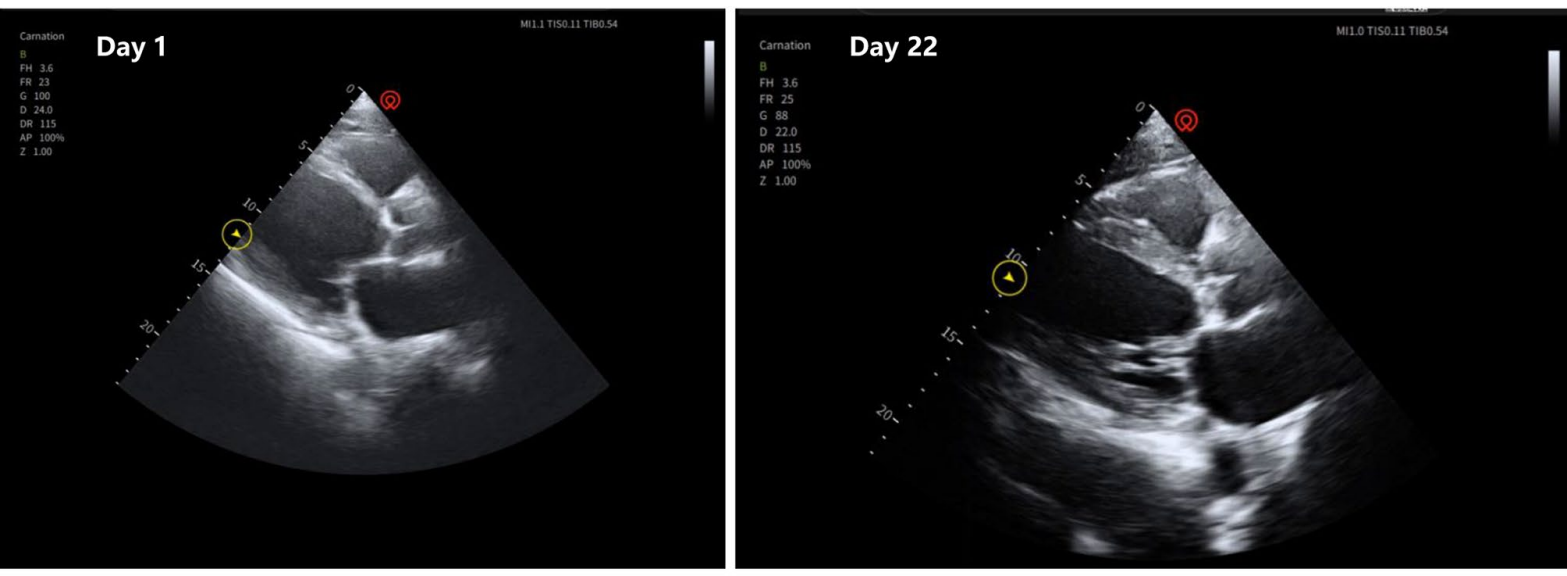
Comparison of echocardiography during the ICU stay. Day 1, the diameters of left atrial and ventricular were 5.7 and 7.0 cm, respectively, LVEF 19%. Day 22, the diameters of left atrial and ventricular were 5.3 and 6.3 cm, respectively, LVEF 32%
